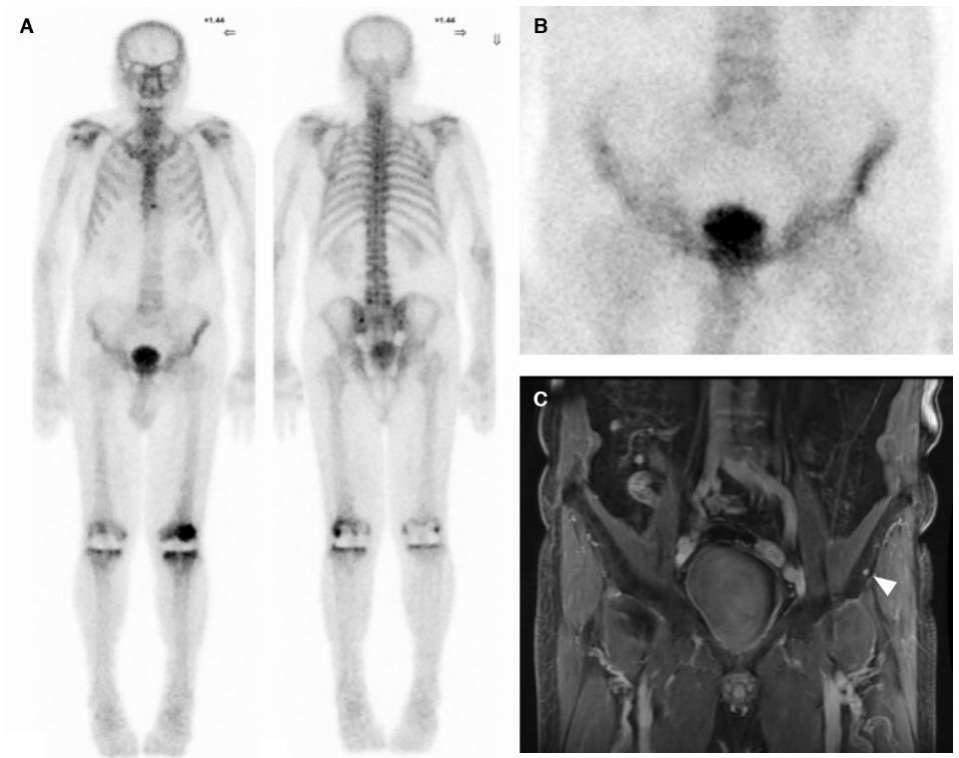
A 78-year-old patient-year-old patient (Gleason score 7(3+4), pT3aN0M0) with BCR (tPSA=0.226 ng/mL) almost 3 years after laparoscopic radical prostatectomy. (A) Bone scan showed no definite evidence of bone metastasis. Areas of increased uptake at the sternum, L5 vertebra, left sacroiliac region, and both knees were most likely due to degenerative or inflammatory processes. (B) Spot view of the pelvis was unremarkable. Prominent uptake at the left iliac spine, relative to the right side, was likely due to asymmetrical positioning. (C) MRI scan of the abdomen revealed a suspicious 0.5-cm lesion at the left iliac bone that was not previously detected on bone scan (white arrow head). This lesion was considered non-specific, and treatment was administered as if no bone metastasis was present
